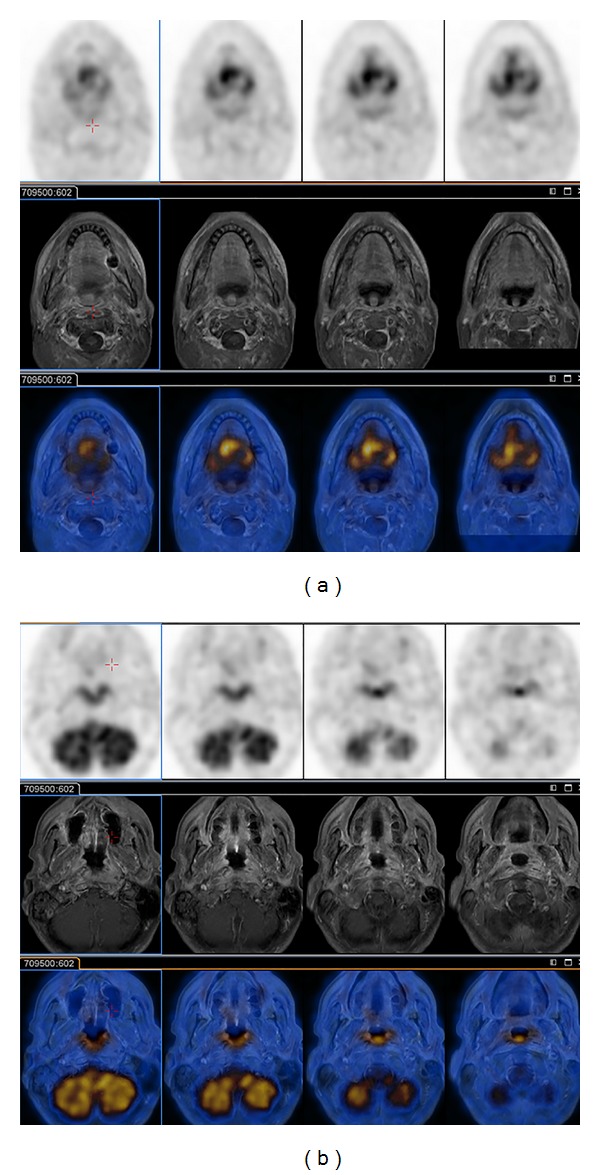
Axial-view images of a patient using a combined PET/MR scanner. The upper rows show PET images of FDG activity corresponding to glucose metabolism. The middle rows show MR images acquired using a T1-weighted, fat saturation sequence. The lower rows show the fused images. The PET images show elevated FDG uptake indicating cancer in (a), the tongue base and (b) the epiglottis.
